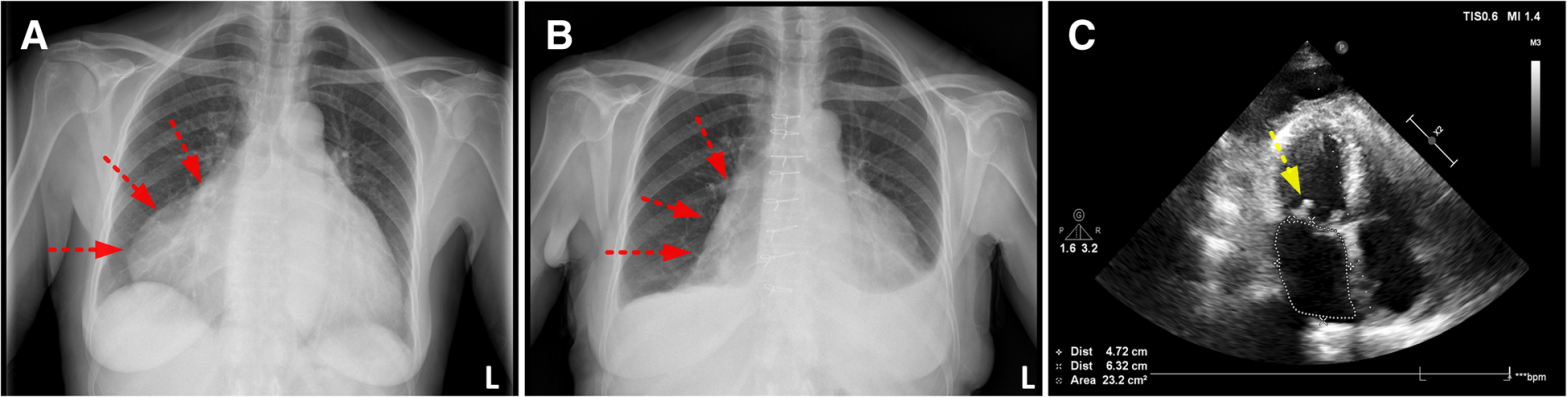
The Radiological examinations of a patient with GLA and right lateral protrusion. Pre- (a) and postoperative (b) AP X-ray images of the cardiac silhouette of a 65-year-old woman with severe mitral regurgitation, pulmonary hypertension, tricuspid regurgitation and dilation of the left atrium (LA area, 81 cm^2^ and a cardiothoracic ratio of 0.8). Red arrows point to the right heart border (a and b). Heart auto-transplantation was performed to replace the mitral valve with a biological prosthesis (yellow arrow) (St. Jude Medical, 27 mm). Excess atrial tissue was surgically removed followed by LA atrioplasty. Red arrows point to the right-lateral lining of the cardiac silhouette (a and b). The procedure successfully restored normal concave left heart border (b), (LA area, 23 cm^2^ and a cardiothoracic ratio of 0.6). Postoperative echocardiogram displayed a normalised left atrium area (indicated by the dotted line) and other chambers without significant morphological distortions (c)
